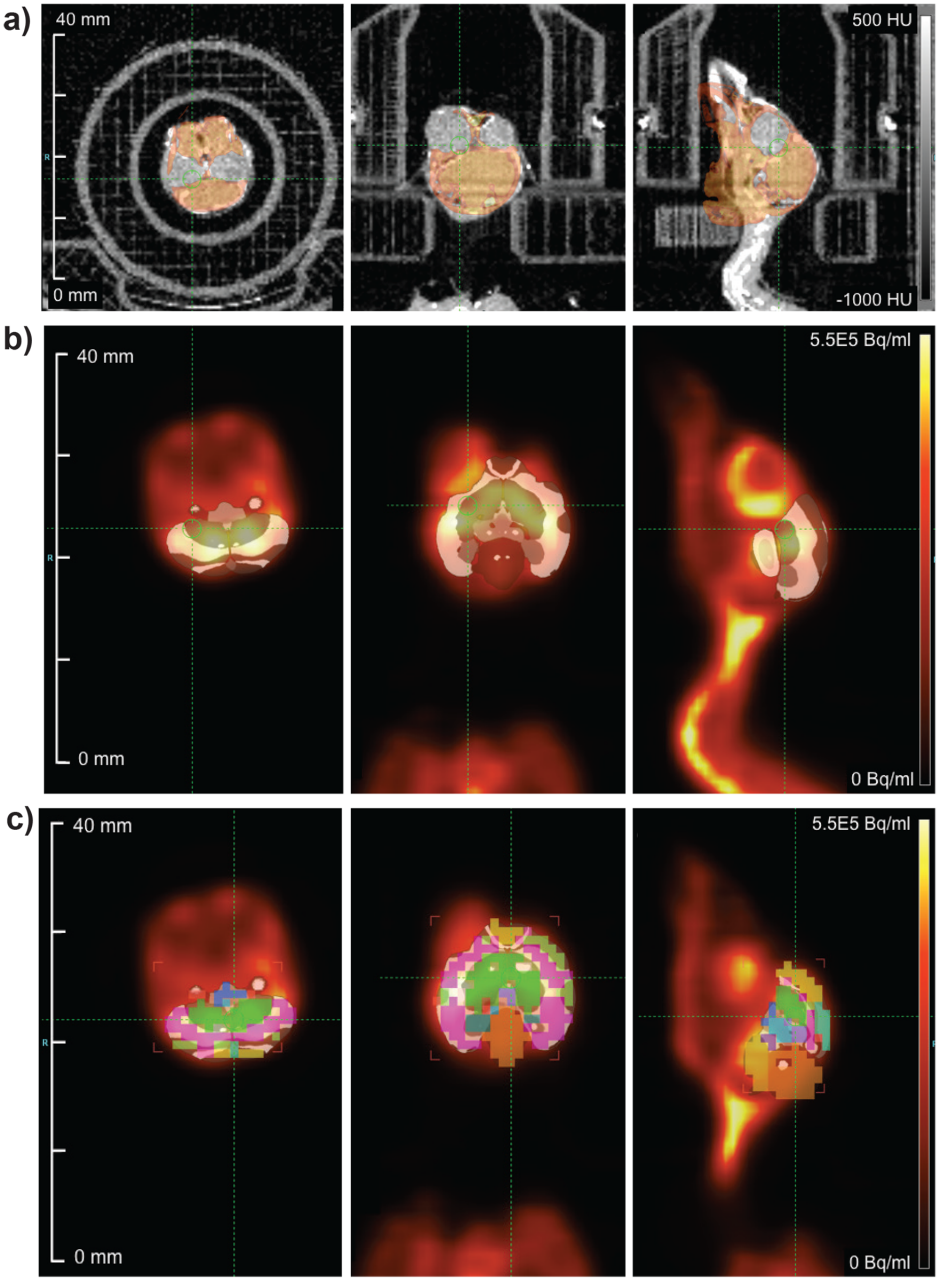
Positron emission tomography (PET) images of female house sparrow brain uptake of ^18^F-fluorodeoxyglucose. Images were processed as follows: a) Computed tomography (CT) images from a published canary atlas were registered with house sparrow CT images using software registration tools. b) Canary atlas regions of interest were transformed to the co-registered sparrow CT and positron emission tomography (PET) images. c) Sparrow regions of interest were created using regions of interest from the canary atlas.
